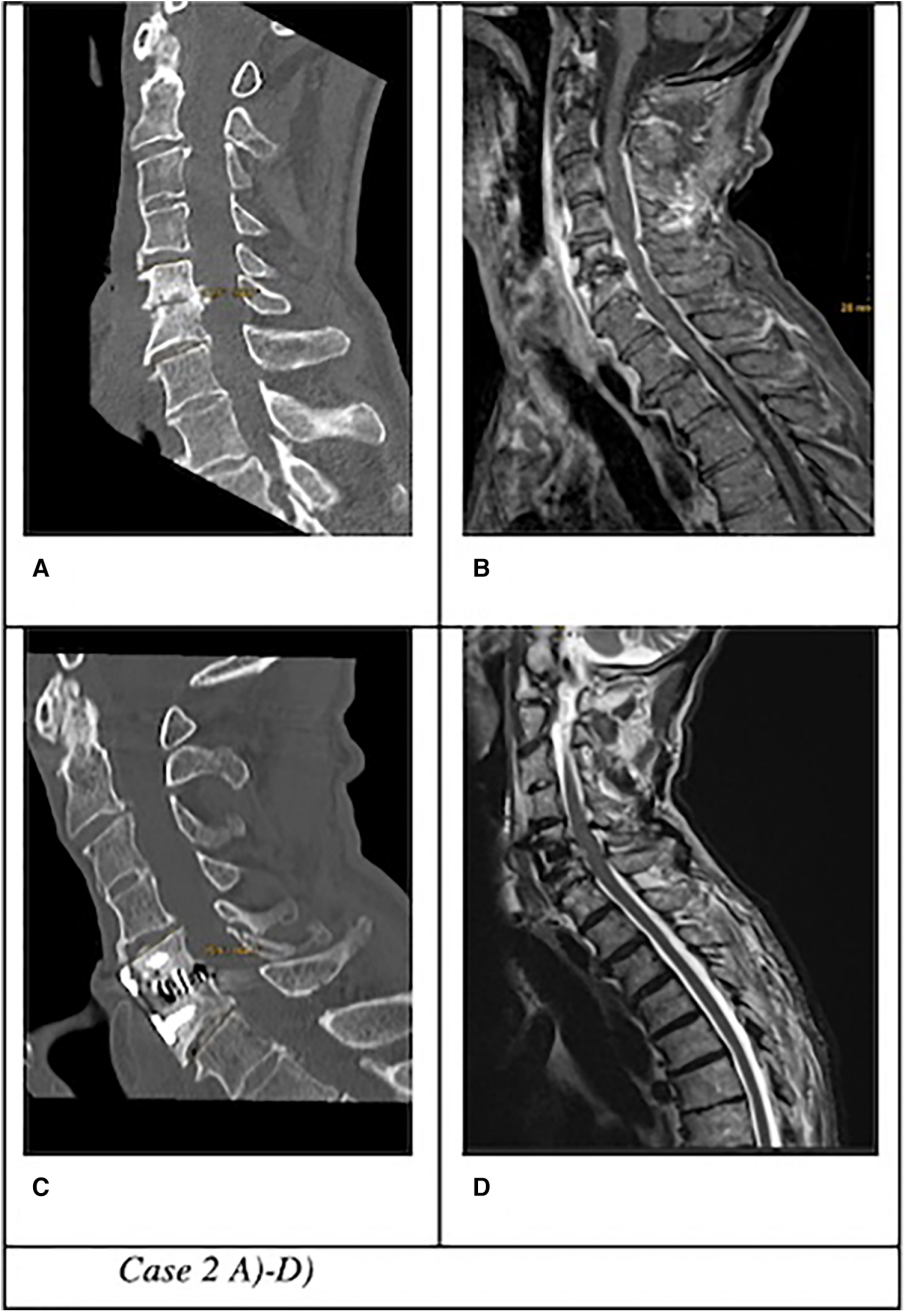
Case 2: Uncomplicated cervical spondylodiscitis at C5/6 in a patient with Parkinson's disease, local sagittal angle of 16°, no epidural abscess. (A) CT and (B) MRI of an 80-year-old patient with swallowing problems, fever, and elevated CRP markers treated with ACDF with anterior plating. (C) CT and (D) MRI on follow-up 3 months after surgery with bony fusion and no signs of infection.
